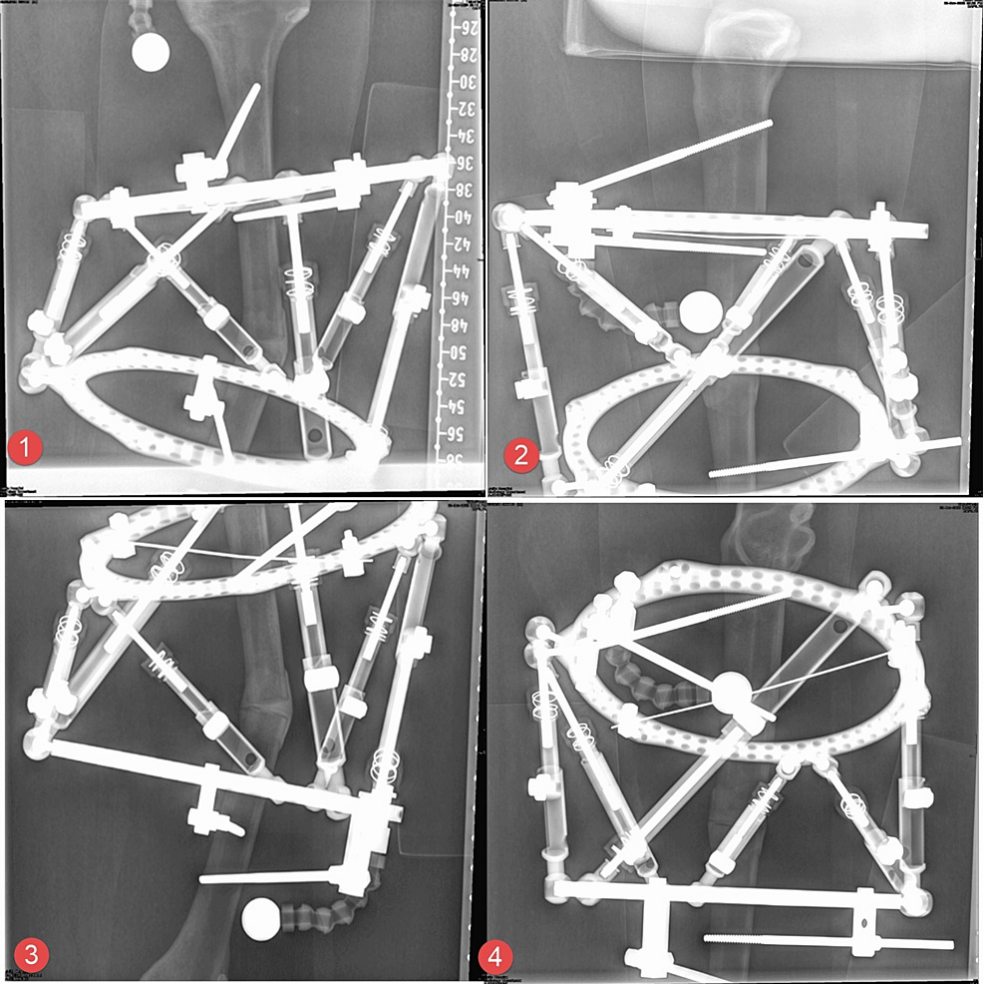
Orthogonal radiographs that are used for deformity quantification and planning
